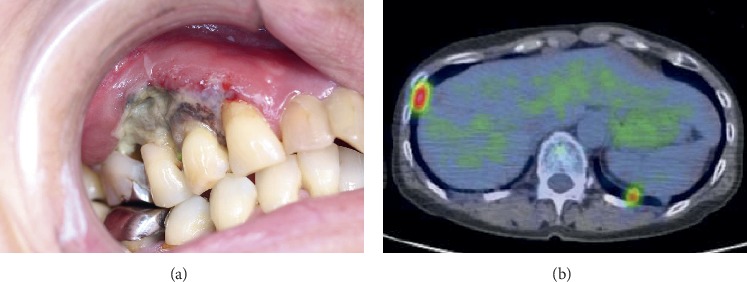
(a) Intraoral photograph: a painful necrotic ulcer in the right maxillary gingiva. (b) Horizontal FDG-PET/CT: pathological uptake of FDG at the bilateral lung.
